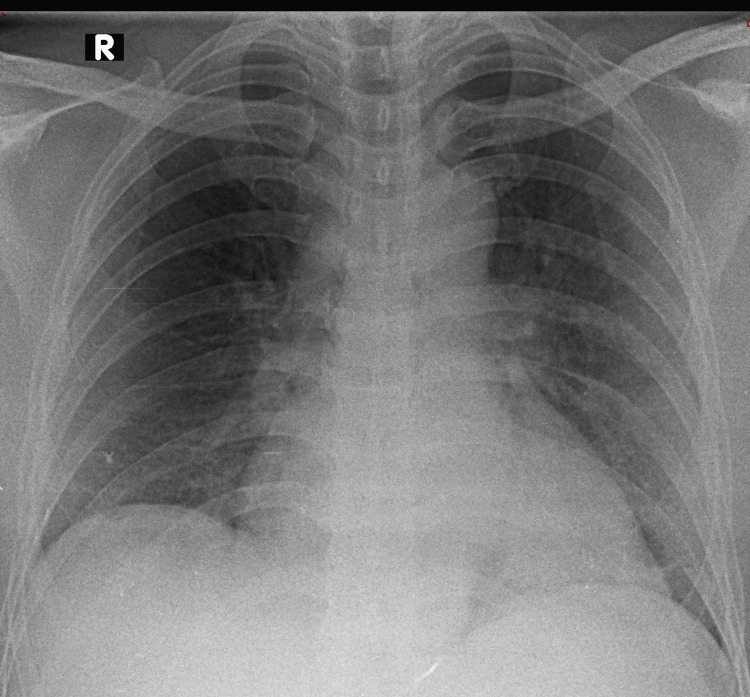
Typical radiographic pattern in a moderate SARS-CoV-2 pneumonia case This CXR showed reticular, ground-glass opacities and consolidations in bilateral lower and mid-zones. In the bilateral mid-zones, the lesion had a peripheral predominant distribution. The Brixia score was 9, the RALE score was 5, and the mCXR score was 7. This case was classified as moderate pneumonia based on clinical severity. The qCSI was 4.
